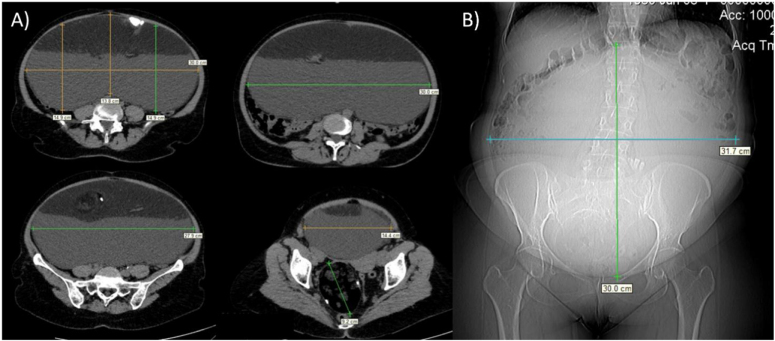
CT scan images of Case 1. (A) Abdominal transverse CT scan showed the left cyst measuring 317 × 300 mm with its liquid component and the right cyst measuring approximately 90 × 50 mm; (B) Longitudinal scan showed the hugest cyst measuring 317 × 300 mm extending from the pelvis to the mesogastrium.
